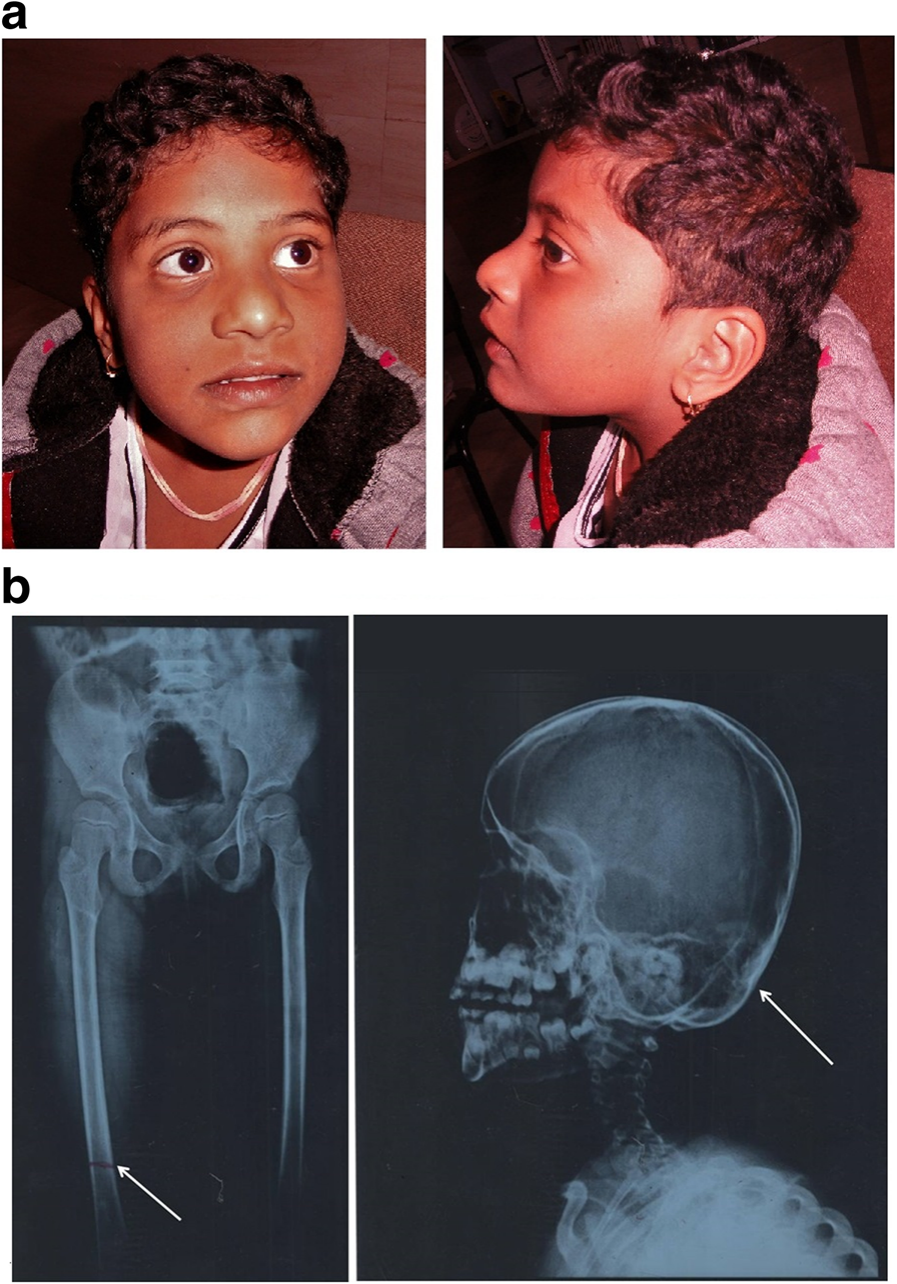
a Clinical picture of the proband showing features of microcephaly, narrow bifrontal diameter, flat forehead, epicanthal folds, hypertelorism, depressed and low nasal bridge with bulbous nasal tip, flaring nares, prominent philtrum and pointed chin. b X-ray of extremities of the proband (Left): the arrow indicates small sclerotic areas observed in lower part of the right femur; X-ray of the skull (Right): abnormal skull shape skull; the arrow indicates minimal sclerosis of lower occipital region-bones
